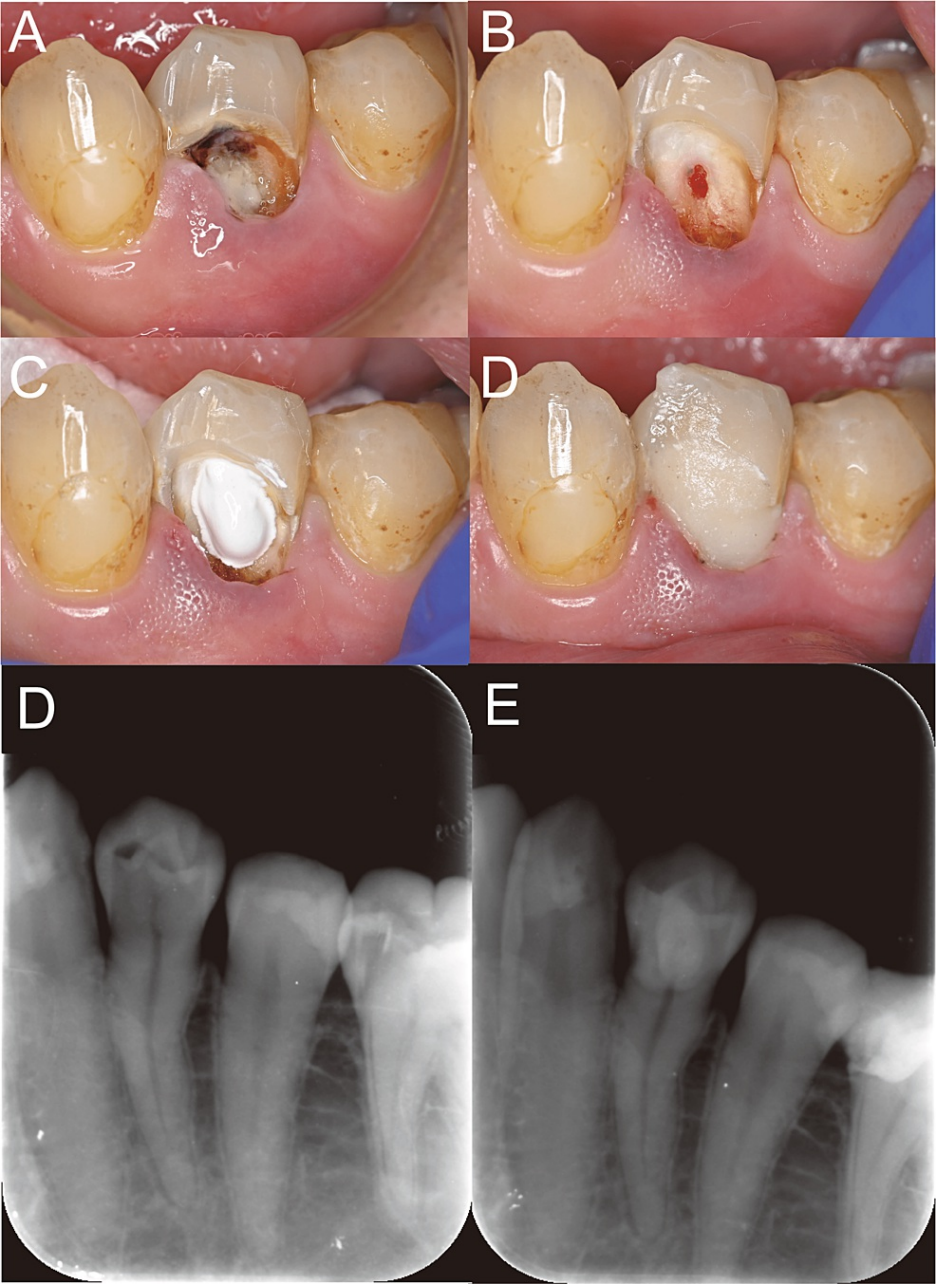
Clinical images and intraoral periapical radiographs of Case one. (A) Before treatment. (B) After removal of dental caries. (C) After placement of mineral trioxide aggregate cement over the dental pulp. (D) Preoperative radiograph. (E) Radiograph taken three months postoperatively.
